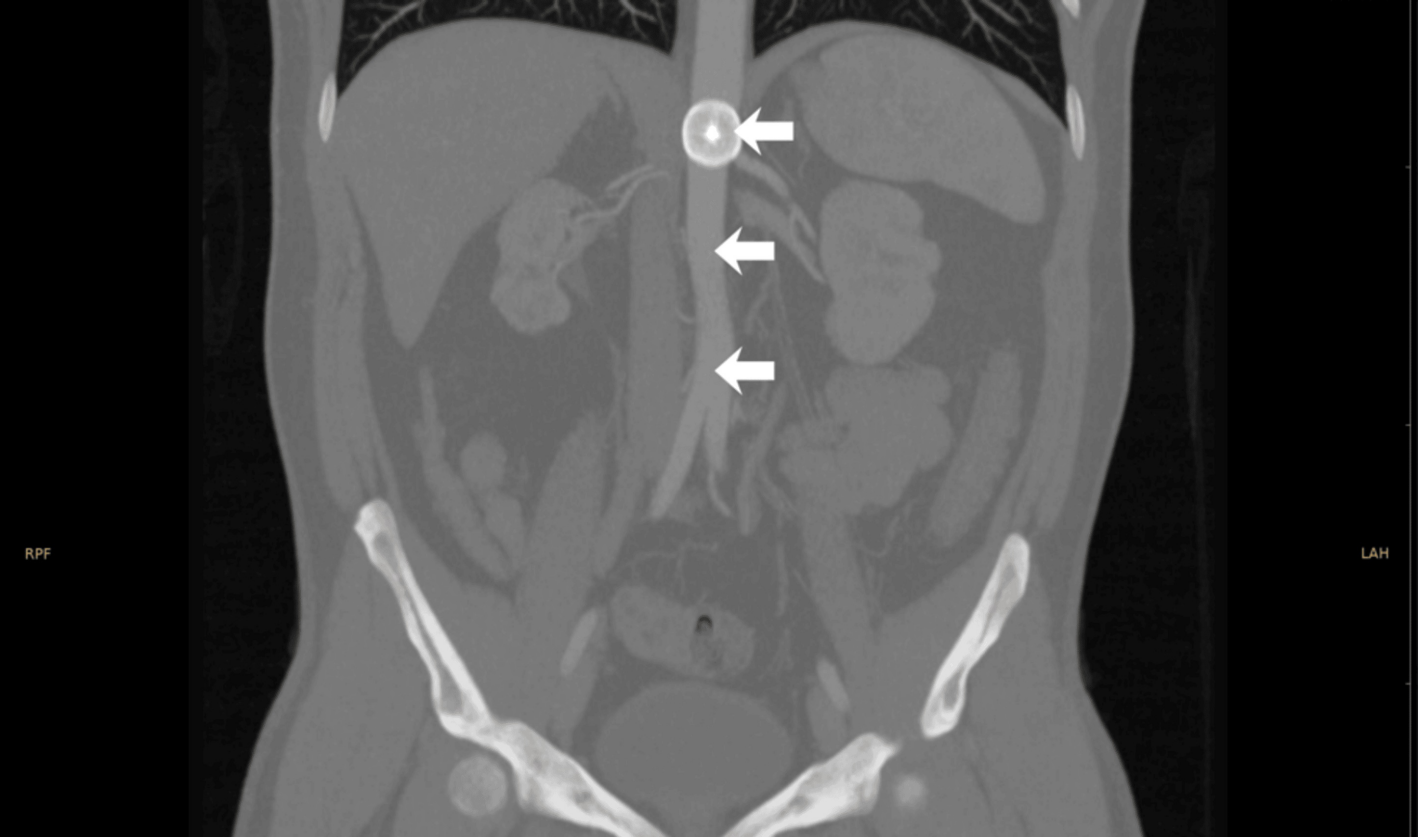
CT angiography demonstrating device embolization into the abdominal aorta Arrows indicate device embolus
